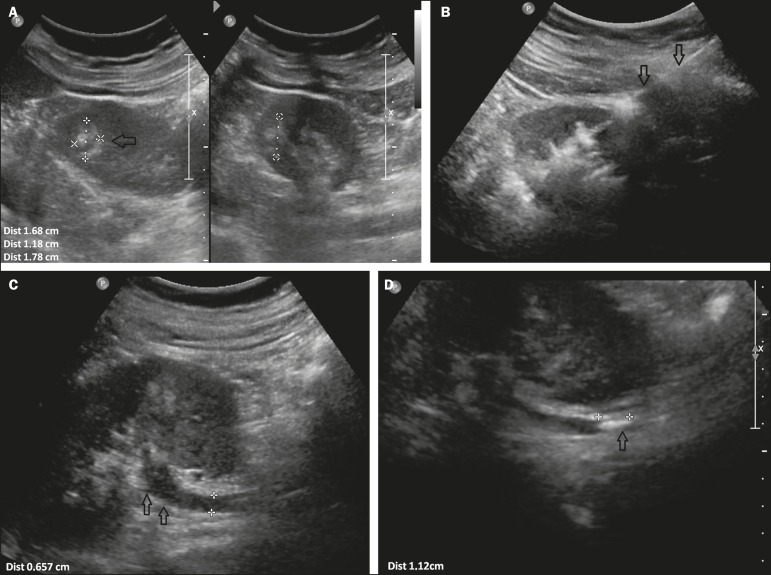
A: Renal ultrasound showing a nodule (arrow) in the middle
